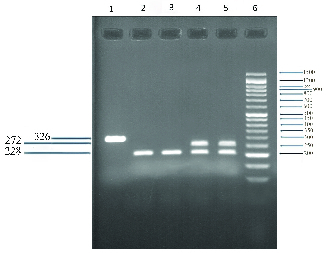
Genotyping of the *PTPN22* 1858 polymorphism by PCR-RFLP. Lane 6: 50bp DNA ladder, Lane 5,4: Samples with heterozygous patterns (CT 272 & 228 bp), Lane 3,4: Samples with homozygote pattern (CC 228 bp), Lane 1: PCR product (326 bp).
